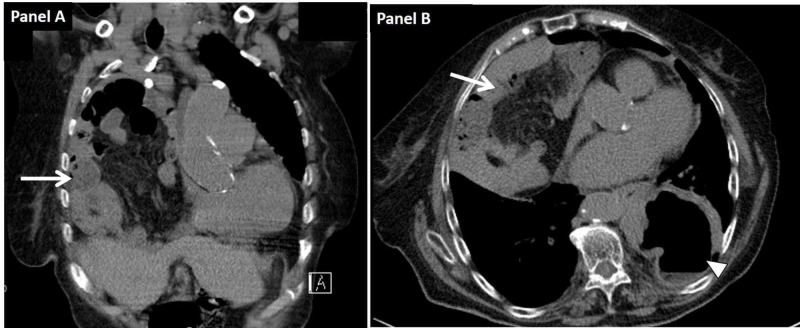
Thoracic computed tomography showing two giant transdiaphragmatic hernias Panel A: Coronal view; Panel B: Transversal view; Arrow: anterior giant paracardiac transdiaphragmatic hernia; Arrowhead: posterior transhiatal gastric hernia
